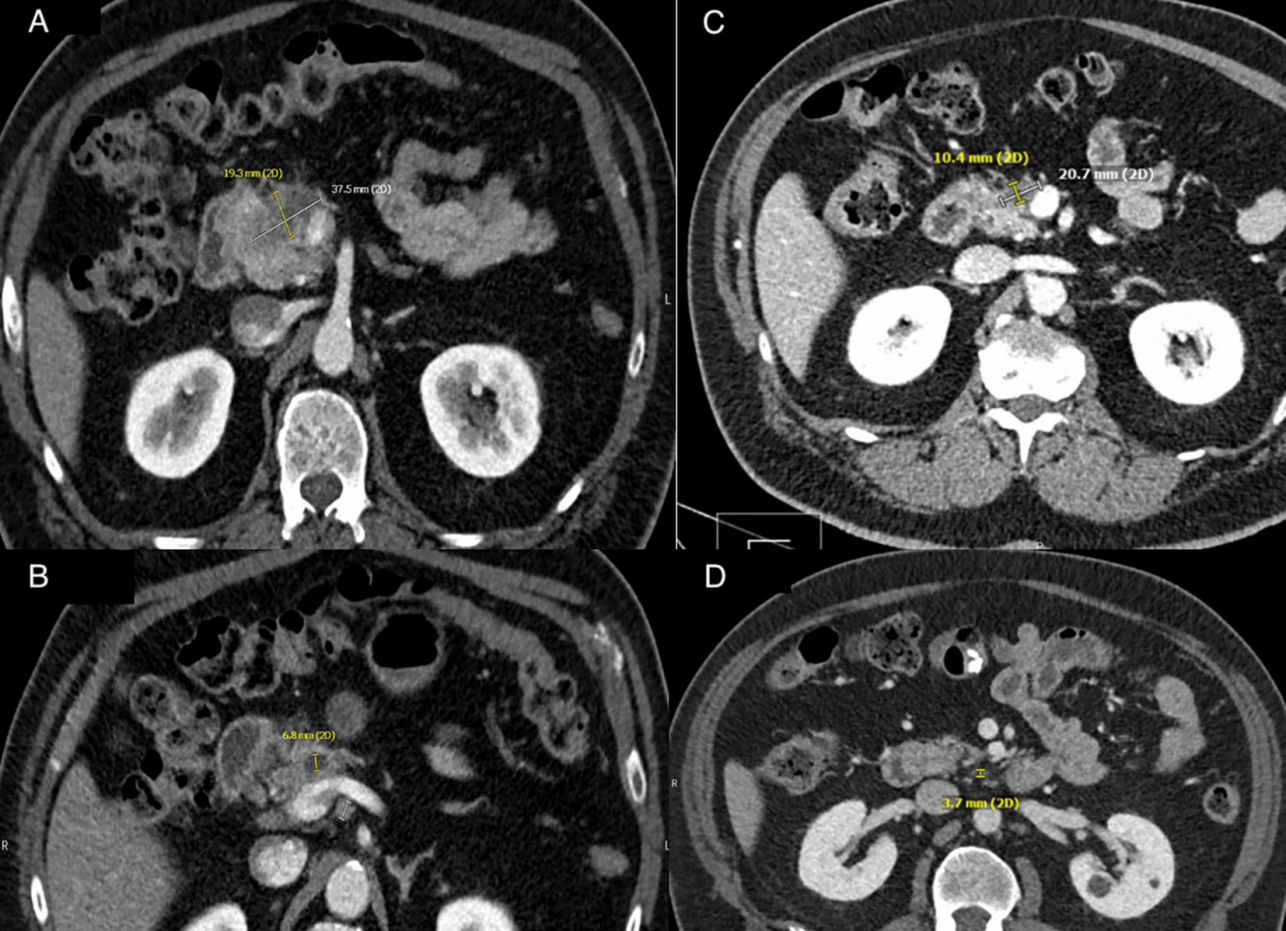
(A) CT abdominal imaging of pancreatic mass at time of diagnosis. A heterogeneous hypodense mass at anterior pancreatic head measuring 3.8 cm × 1.9 cm with associated pancreatic ductal obstruction. (B) CT abdominal imaging of lymphadenopathy at time of diagnosis. Lymph node posterior to the uncinate process measuring 10 mm on axial imaging. (C) CT abdominal imaging of pancreatic mass after five cycles of chemotherapy. Pancreatic mass with interval decrease in size to 2.1 cm × 1.0 cm. (D) CT abdominal imaging of lymphadenopathy after five cycles of chemotherapy. Interval decrease in size of lymph node posterior to the uncinate process measuring 3.7 mm on axial imaging. Created with BioRender.com.
